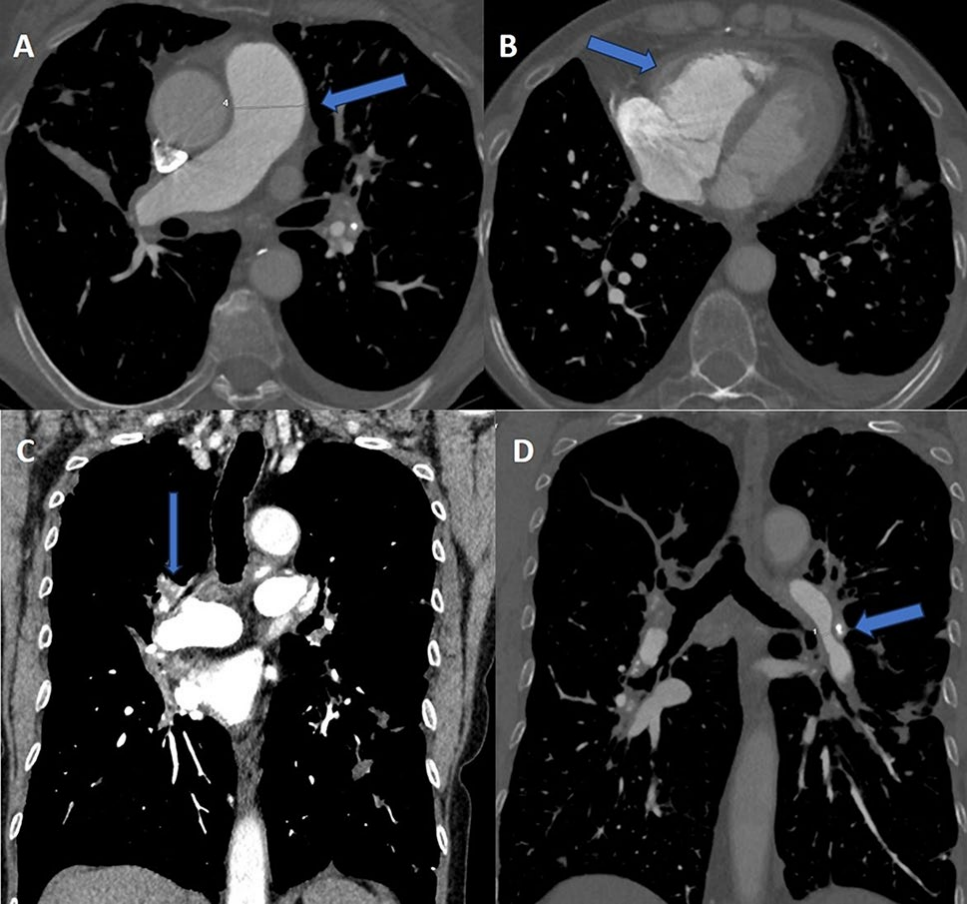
Computed tomography pulmonary angiography (CTPA) a 69-year-old-female with sarcoidosis: (a) axial image, mediastinum window, at the level of the pulmonary trunk shows a dilatation of the pulmonary trunk to 33 mm (blue arrow); (b) axial image at the level of the heart shows enlarged right ventricle (blue arrow) and mild pleural effusion on the left side; (c) coronal image demonstrates complete occlusion of the right upper lobe artery (blue arrow); (d) coronal image reveals stenosis of the left lower lobe artery just below the branch of the artery to segment six with post-stenotic dilatation, and enlarged calcified lymph nodes on this level (blue arrow)
